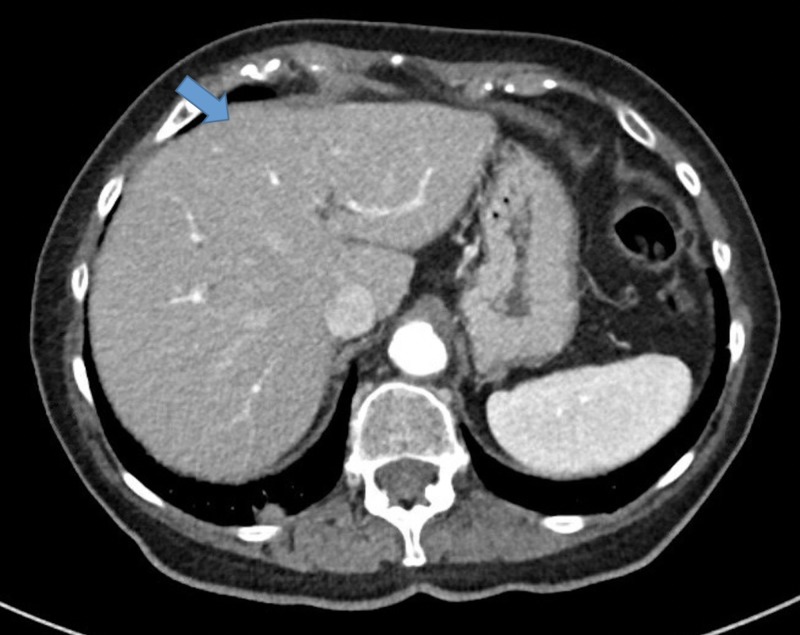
Computed tomography scan of the abdomen after antibiotics showing resolution of the hypodensity in the liver.
